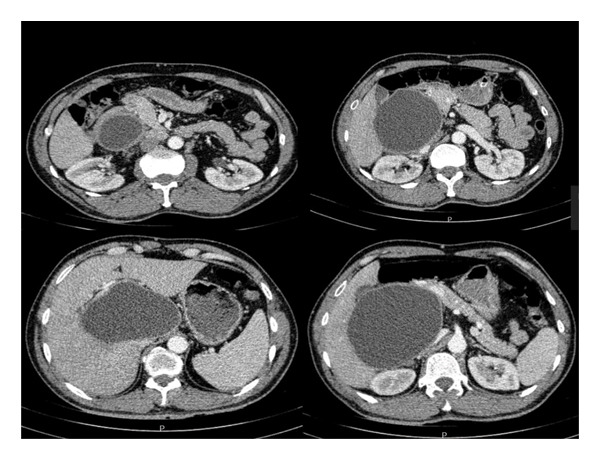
Axial contrast‐enhanced CT images of the abdomen demonstrating a large, well‐circumscribed, unilocular hydatid cyst in the right hepatic lobe. The cystic lesion measures approximately 135 × 103 mm and exhibits a sharply defined wall with homogeneous fluid attenuation, consistent with a CE1‐type cyst according to WHO classification. The lesion exerts a significant extrinsic mass effect on adjacent vascular structures, notably the portal vein and inferior vena cava (IVC), without evidence of intralesional calcification or biliary ductal dilation. No septations, daughter cysts, or signs of intrabiliary rupture are visualized, supporting the diagnosis of a primary unilocular cystic echinococcal lesion.
